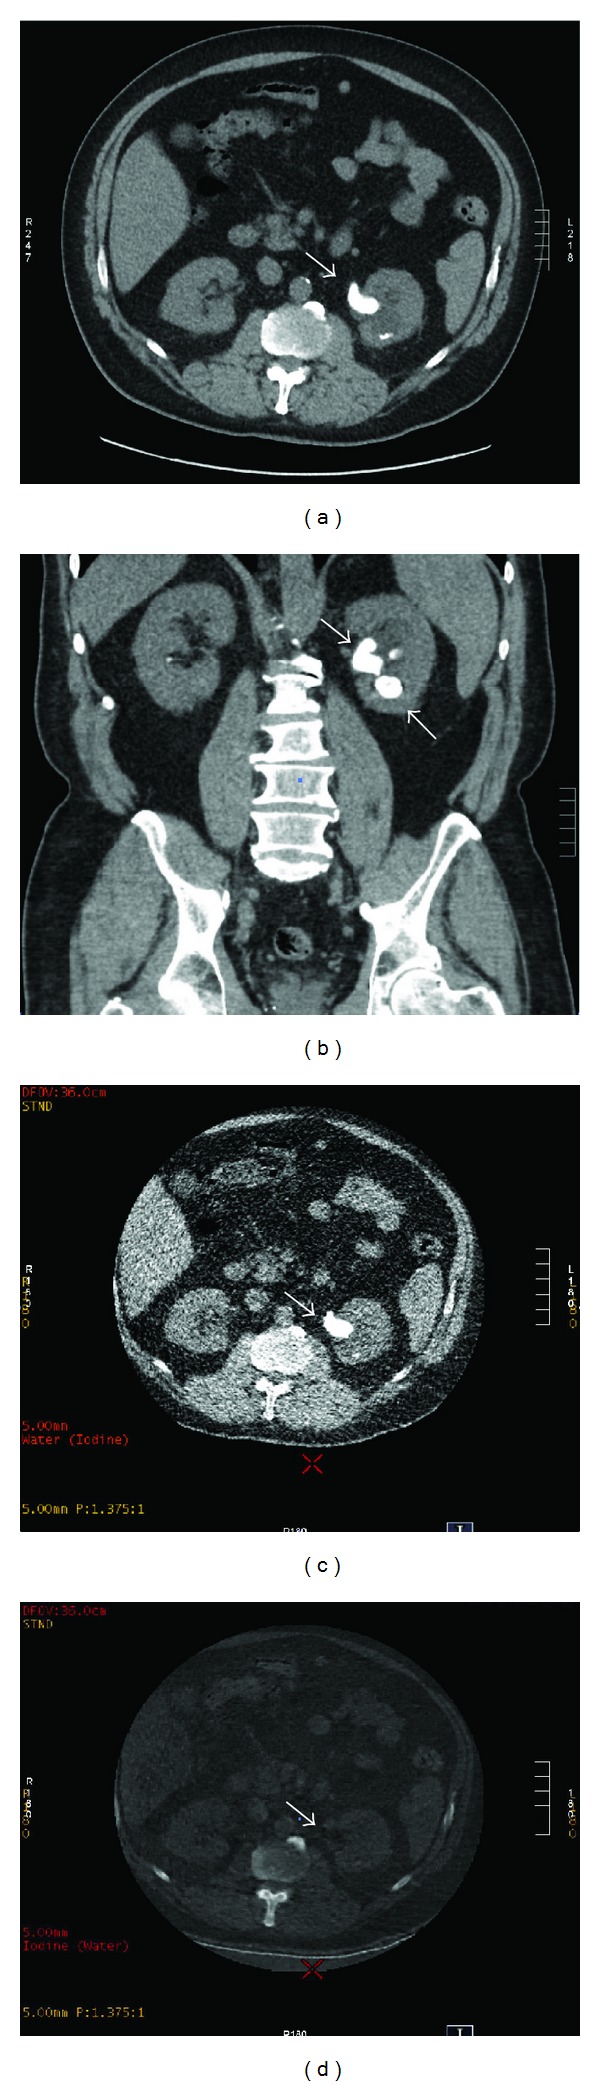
A 42-year-old male with a history of renal calculi presenting with left flank pain. Noncontrast CT for the assessment of renal calculi in axial (a) and coronal (b) reformats. These images clearly show a large calculus in the left renal pelvis extending into the upper ureter as well as a second large calculus in the lower pole of the left kidney (arrows). Axial dual energy noncontrast CT images in the same patient showing iodine- (c) and water- (d) based attenuation. The calculus is visible on the iodine-attenuated image (c) and is unchanged when compared to the standard CT. However, the water-attenuated image (d) shows “dropout,” and the calculus is no longer visible. This indicates the presence of low-molecular-weight elements. Post removal, this stone proved to be predominantly composed of urate.
